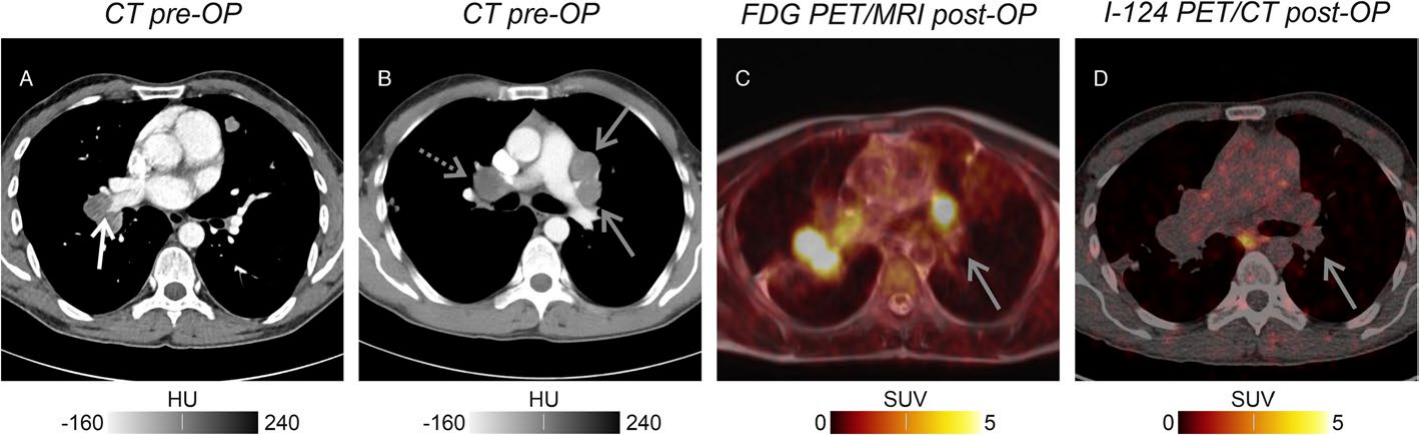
CT pulmonary angiogram (A, B) with exemplarily indicated filling defect of contrast media (white arrow) and bihilar metastases (solid and dashed grey arrows). ^18^F-FDG-PET/MRI (C) and ^124^I-PET/CT (D) after incomplete left hilar metastasectomy showing a partly resected radioiodine-negative left hilar metastasis (solid grey arrows)
